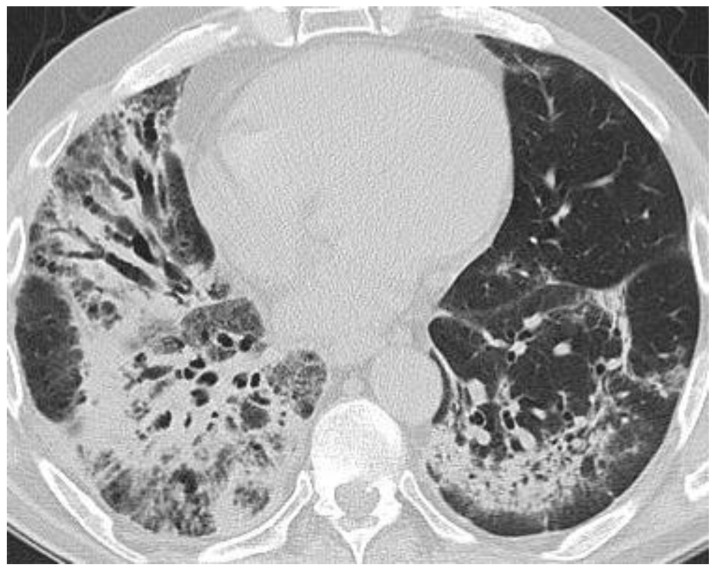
Chest computed tomography scan after 16 weeks of immunological treatment: ground-glass opacities in both lungs, more prominent in right lung.
